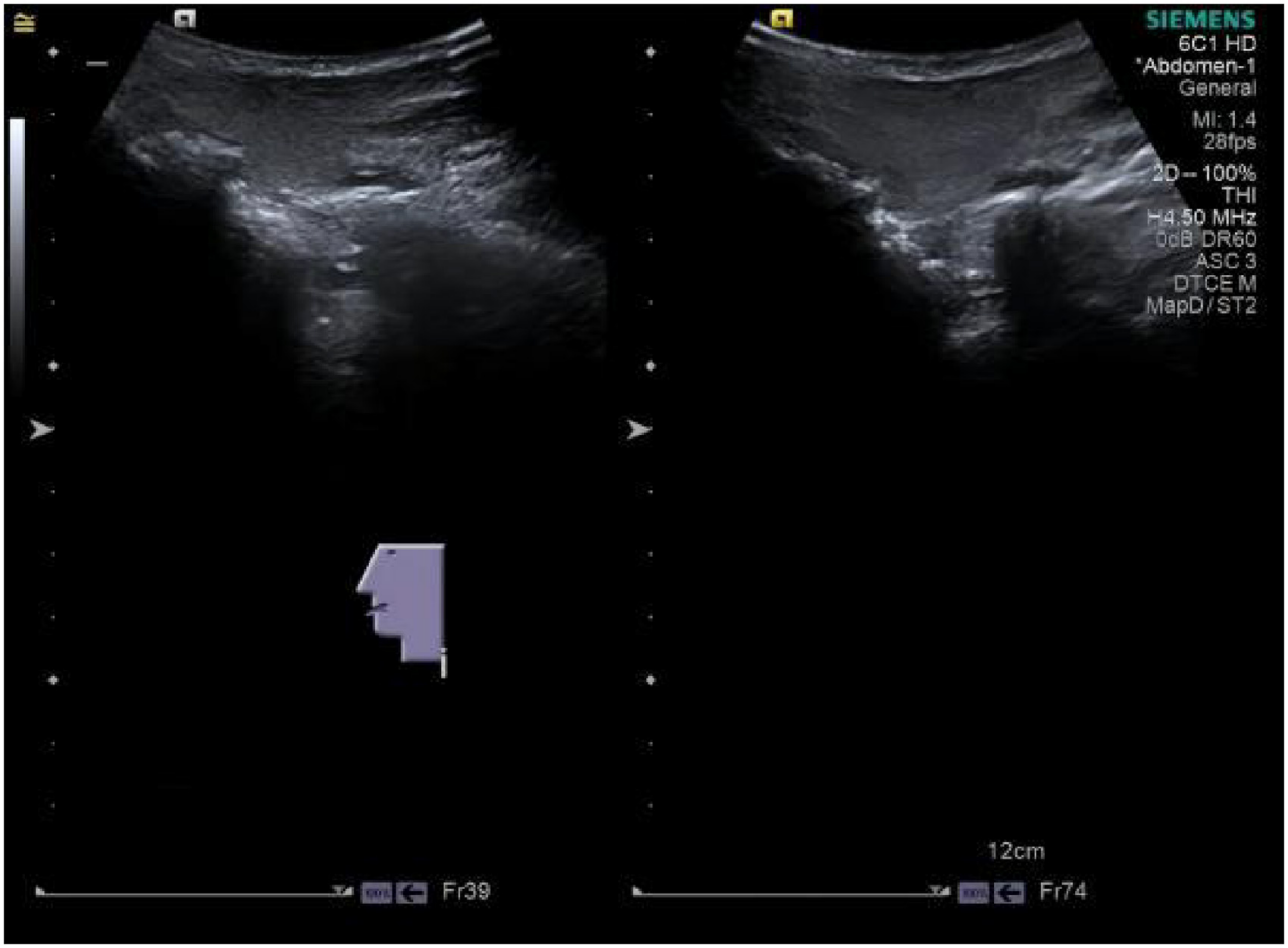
Patient parotid ultrasound.
